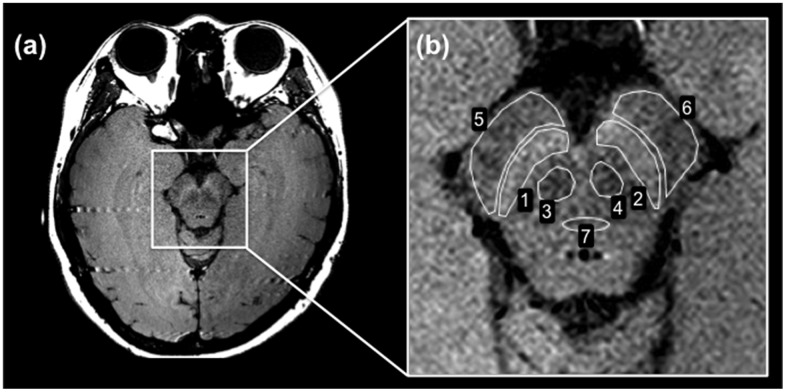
Neuromelanin-sensitive MRI of the lower midbrain. (a) 2D-neuromelanin-sensitive MRI. The SNc appears as the high SI area. The SCP is the small low SI area below the SNc. The CP is above the SNc. The MT is located in the middle of the lower midbrain. (b) The ROIs for measuring the SI were traced manually at the following locations on a single slice: SNc (ROIs 1 and 2), SCP (ROIs 3 and 4), CP (ROIs 5 and 6), and MT (ROI 7).
